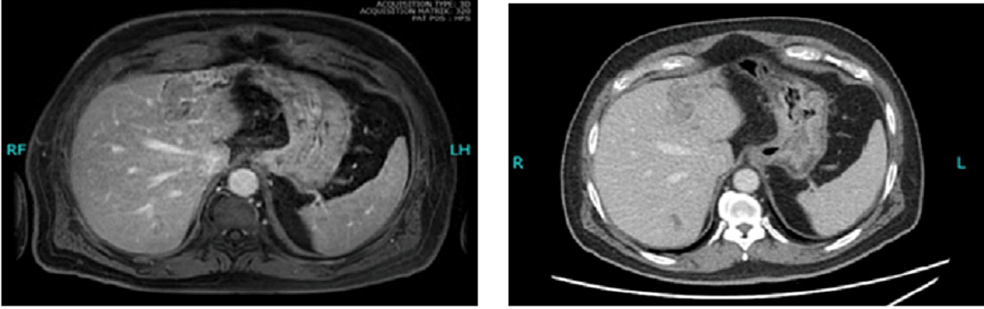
Contrast-enhanced CT showing a heterogeneous infiltrative area involving the left liver lobe in post-contrast (A) and delayed (B) phases.
